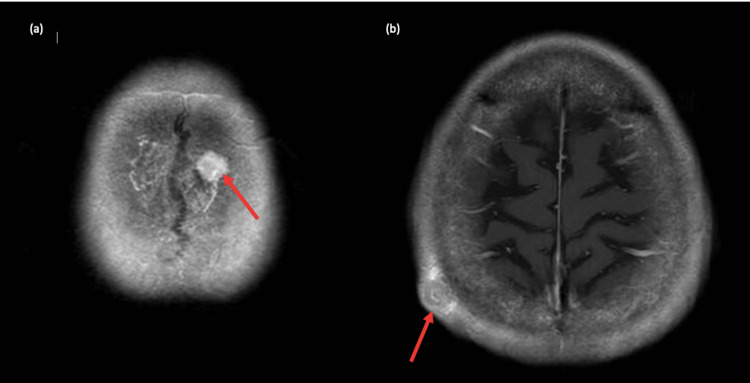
MRI brain: (a) intradiploic metastatic lesion; (b) right parietal metastatic lesion. MRI, magnetic resonance imaging
